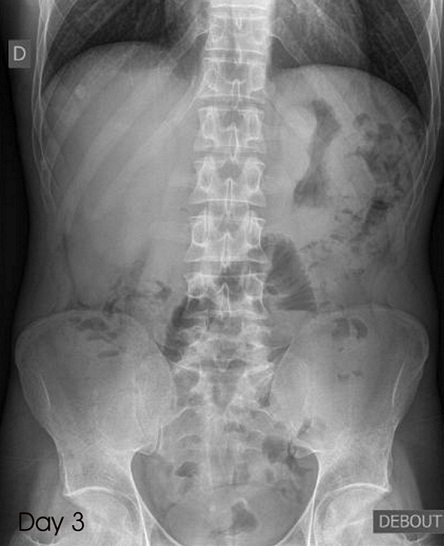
Plain abdominal X-ray after 3 days
